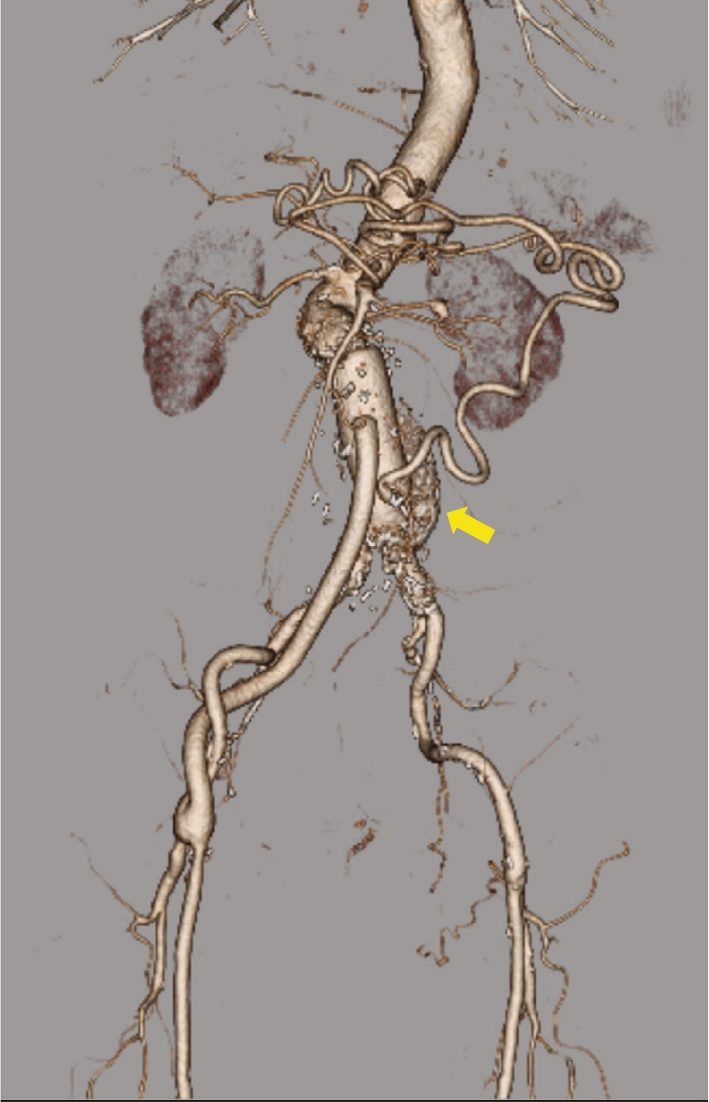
Postoperative contrast-enhanced computed tomography reveals the patent inferior mesenteric artery (arrow).
